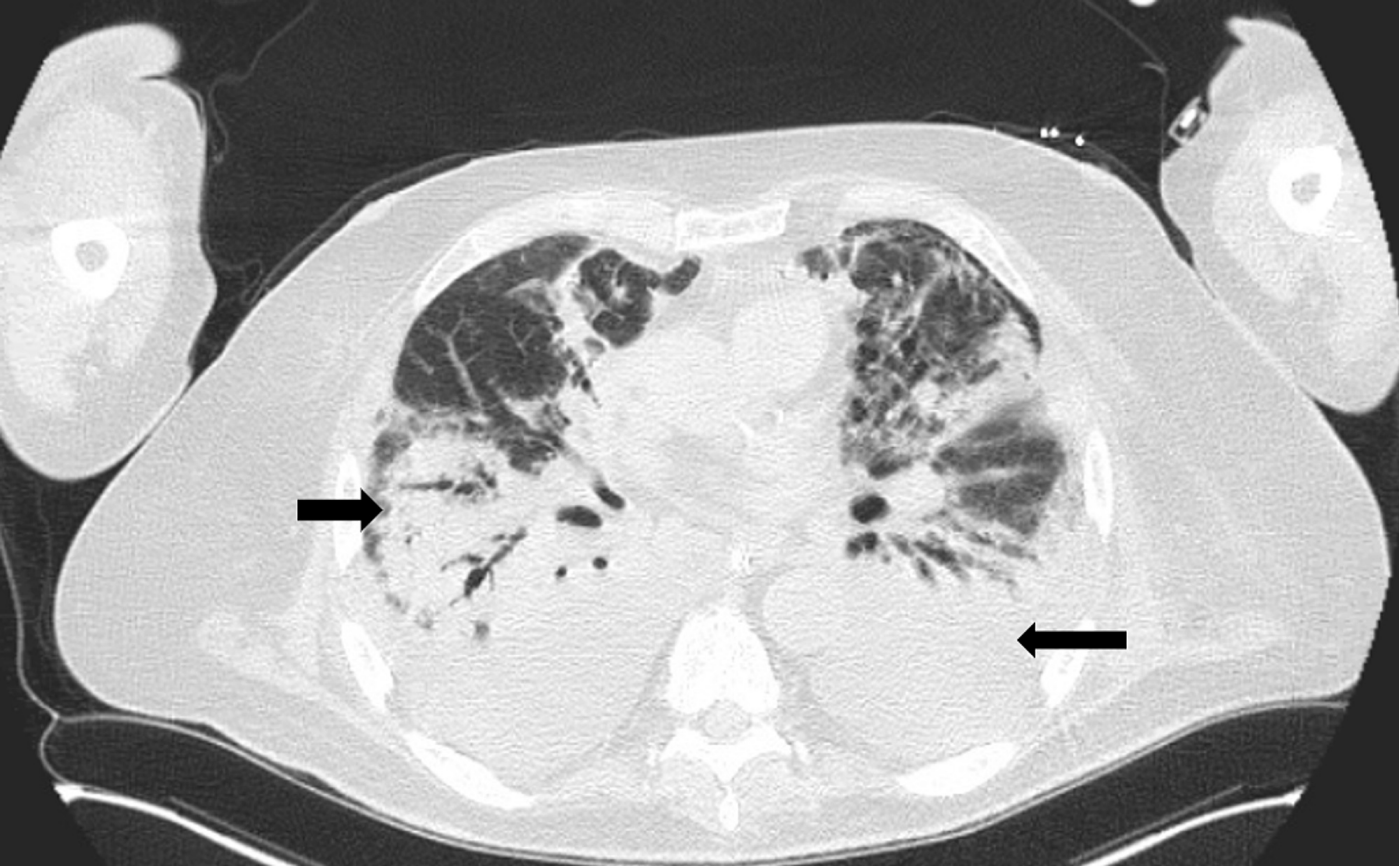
CT chest shows multilobar diffuse consolidations as marked by black arrows
